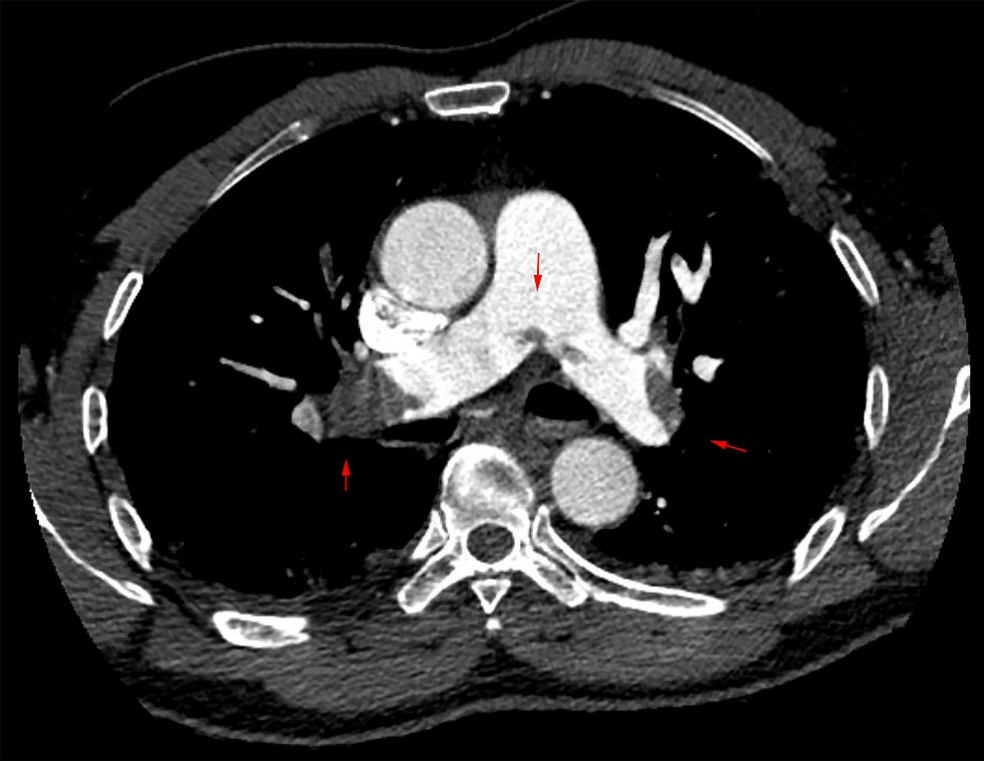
CT pulmonary angiogram. Presenting CT pulmonary embolism (PE) showing saddle pulmonary embolus in the axial view. Red arrows point to filling defects seen in the main pulmonary arteries and also at the bifurcation.
